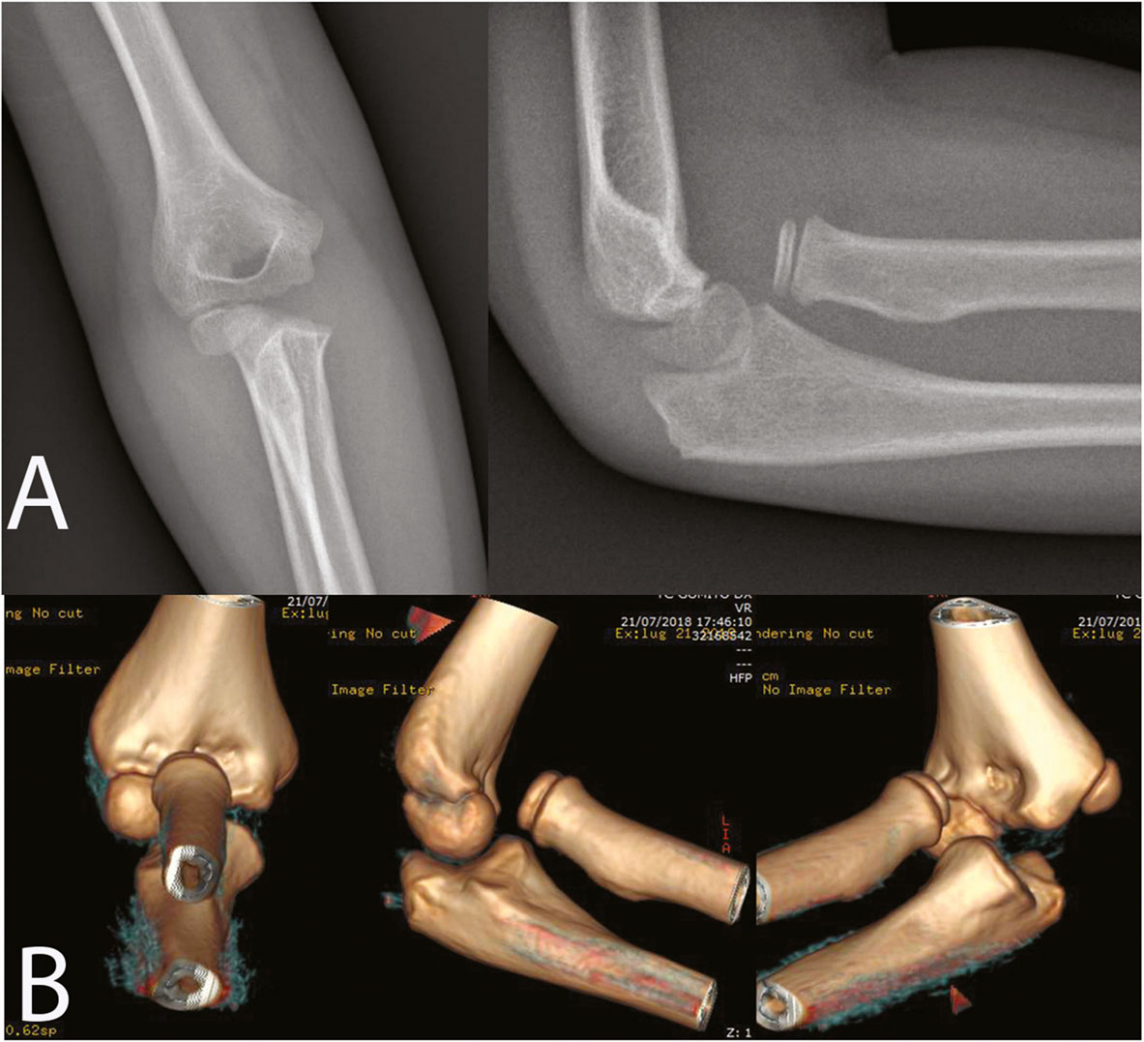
X-ray (a) and CT scan (b) of radial head dislocation
